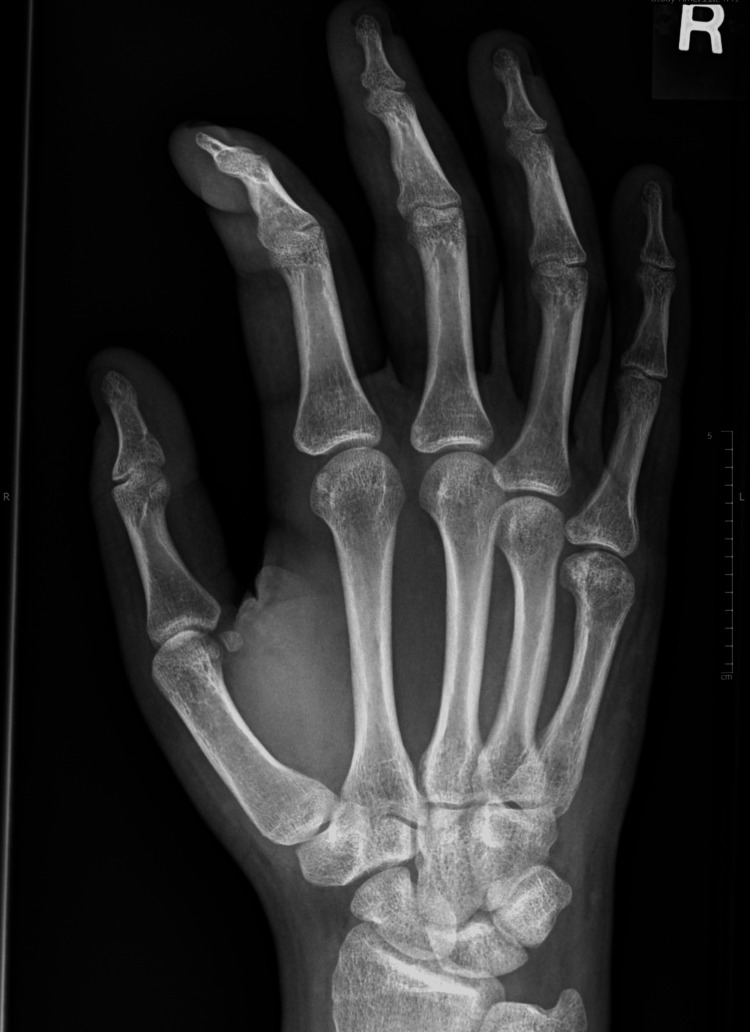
The patient's radiograph taken 12 weeks following removal of the fixator.
